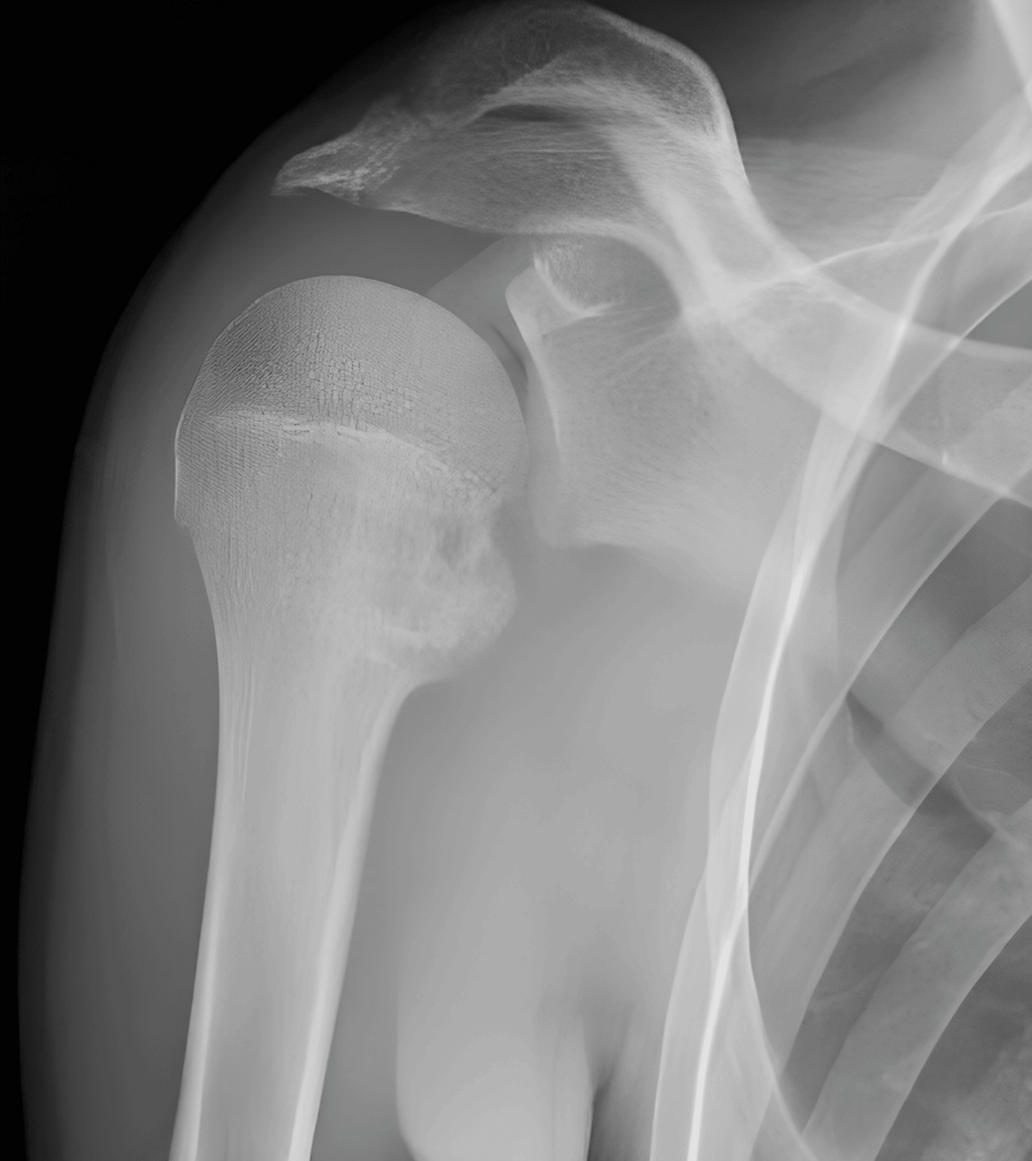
Plain radiography confirming bone union at four months postoperatively
